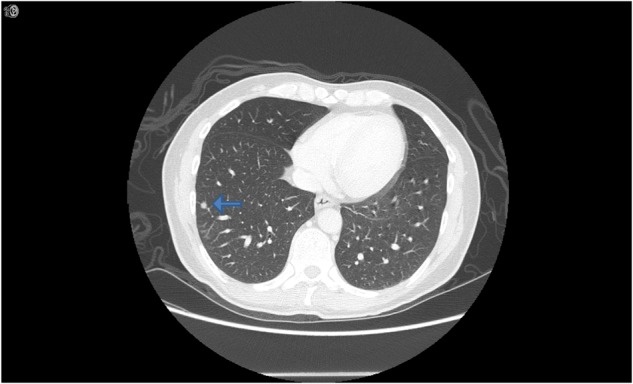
CT thorax image of case 1. Nodule seen in peripheral right lung field.
